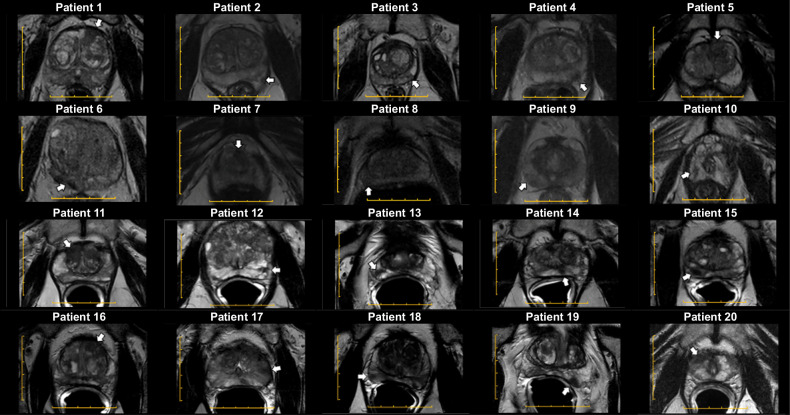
Prostate cancer lesions at baseline on the T2-weighted magnetic resonance image from patients on active surveillance. Prostate cancer lesions on the T2-WI from patients on active surveillance at University College London Hospitals (Patients 1–10) and Ospedale San Raffaele (Patients 11–20). White arrows point to the lesion of interest (the index lesion) for each subject, and the scale in each scan is 5 mm in length
